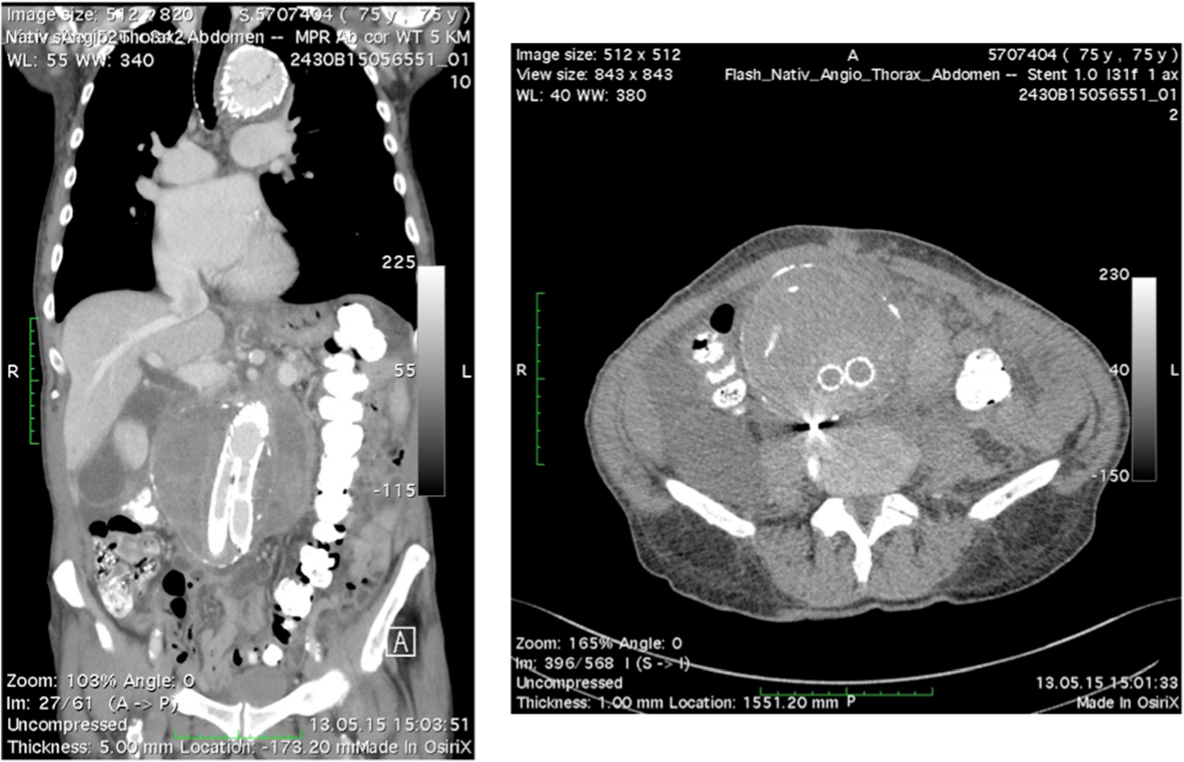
CT showing 10x9.5 cm infra-renal aortic aneurysm with a new para-aortic hematoma
